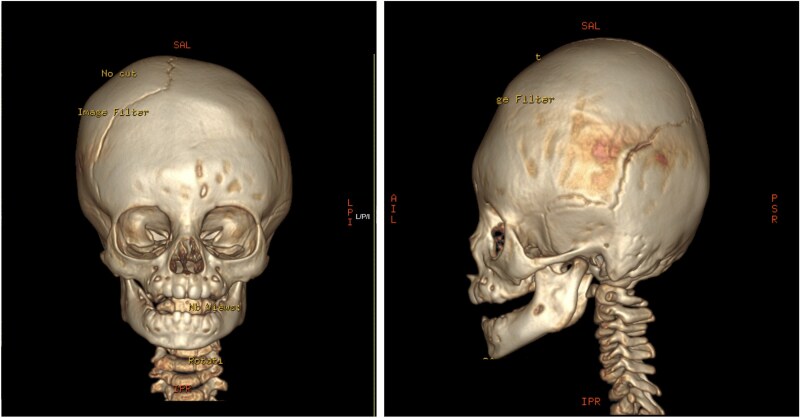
Pre-operative 3D reconstruction CT showing left plagiocephaly with towering, and a subtle harlequin sign.
